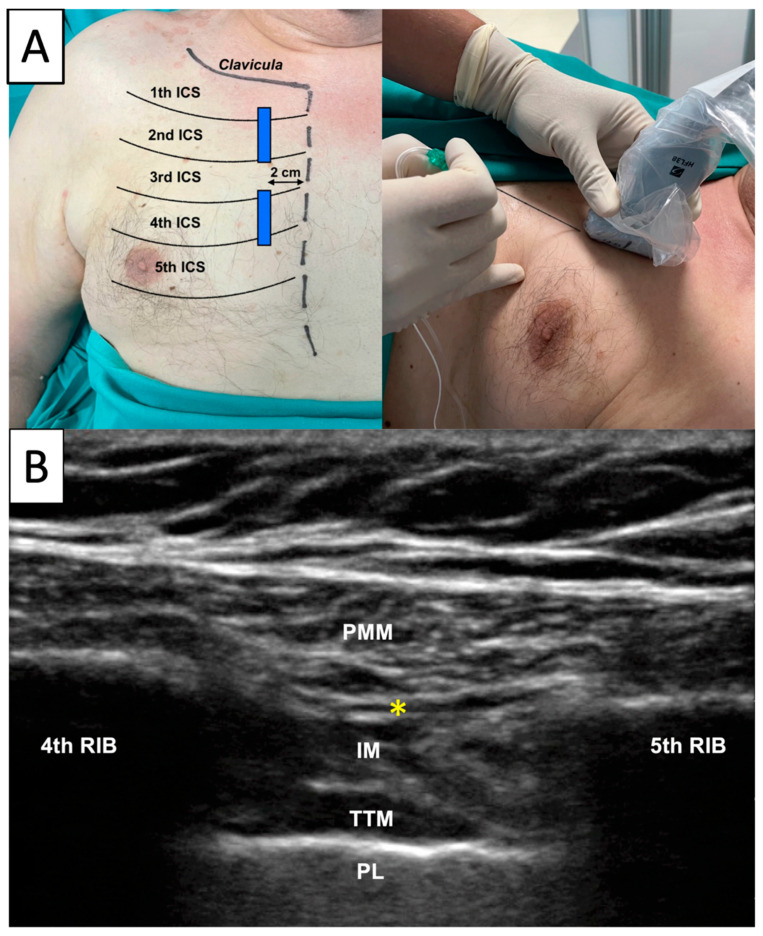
Parasternal Block Execution. *Legend:* A high frequency ultrasound probe was positioned immediately lateral to the sternum identifying the second and the fourth intercostal spaces (A). Then, an echogenic 100 mm needle (Stimuplex ultra-360, BBraun Deutschland GmbH & Co., Melsugen, Germany) was advanced through the skin with an in-plane approach and an injection of 10 mL 0.5% ropivacaine was performed between pectoral major and intercostal muscles for every intercostal space, bilaterally, with a total dose of 200 mg of ropivacaine (B). PMM: pectoral major muscle; IM: intercostal muscles; TTM: transversus thoracis muscle; PL: pleura; *: point of injection.
